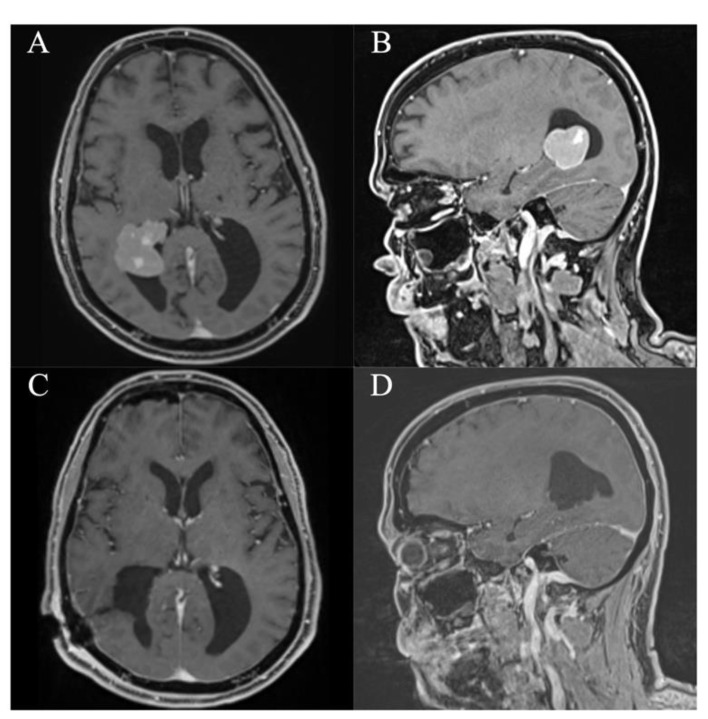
Case 3. Axial and sagittal MR images, T1-weighted sequences with contrast medium preoperatively (A,B) and immediately postoperatively (C,D) after resection of the intraventricular meningeoma via a right parietal endoscopic-assisted approach.
